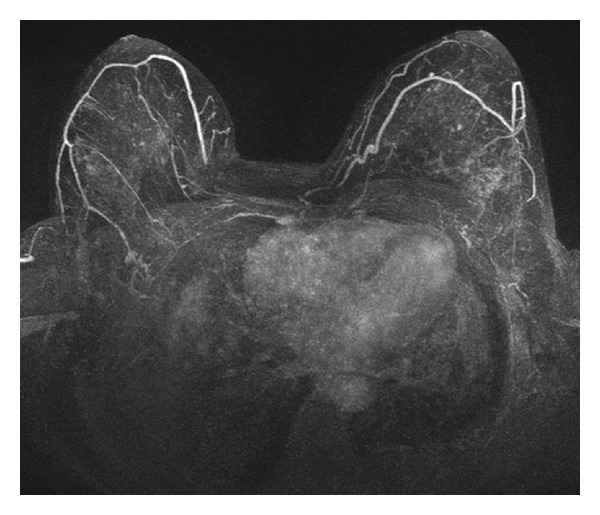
Axial 3D maximal intensity projection of enhanced breast MRI with fat suppression shows diffuse nonmass infiltrating enhancement symmetrically in bilateral breast parenchyma.
